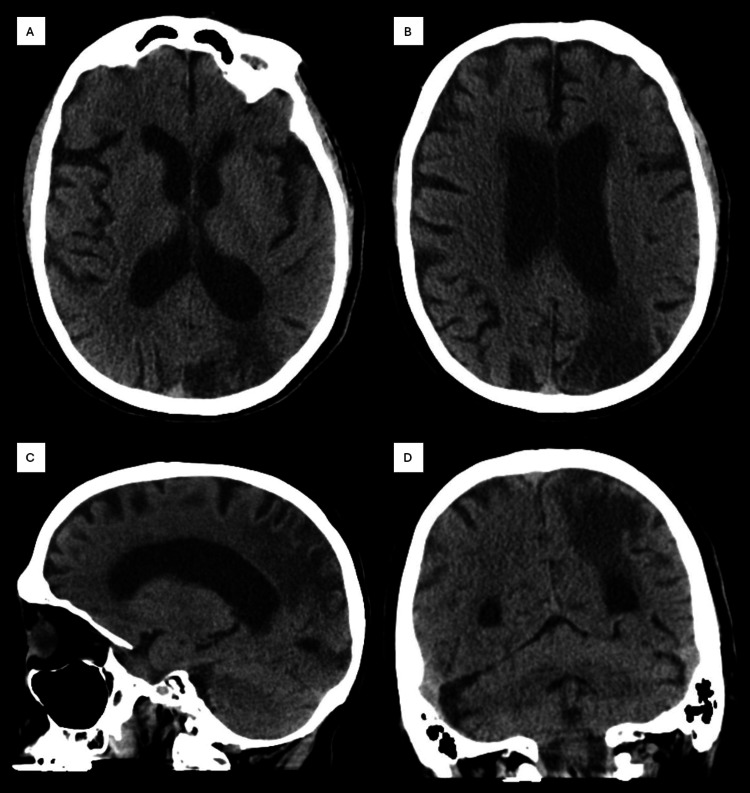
Non-contrast CT of the head obtained in the ED. Non-contrast CT scan of the head in the axial (A and B), sagittal (C), and coronal (D) views. Findings showed no evidence of intracranial hemorrhage and a remote infarct in the left posterior parieto-occipital region. CT, computed tomography; ED, emergency department
